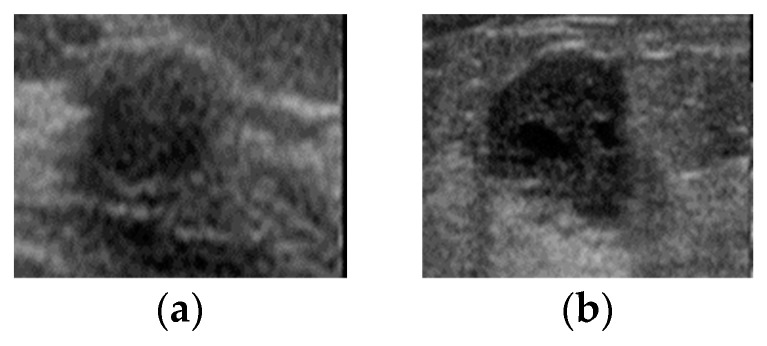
Representative images from Mendeley breast ultrasound dataset: (a) benign; (b) malignant.
